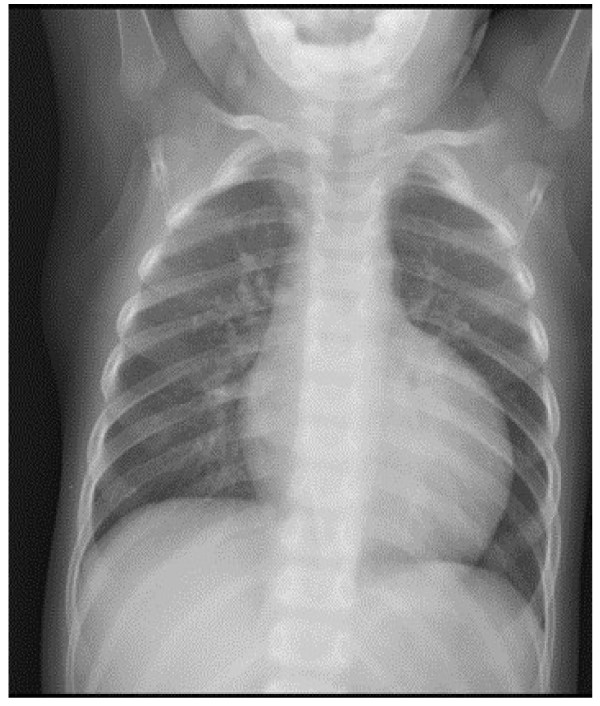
Chest X-ray showing severe cardiomegaly.
